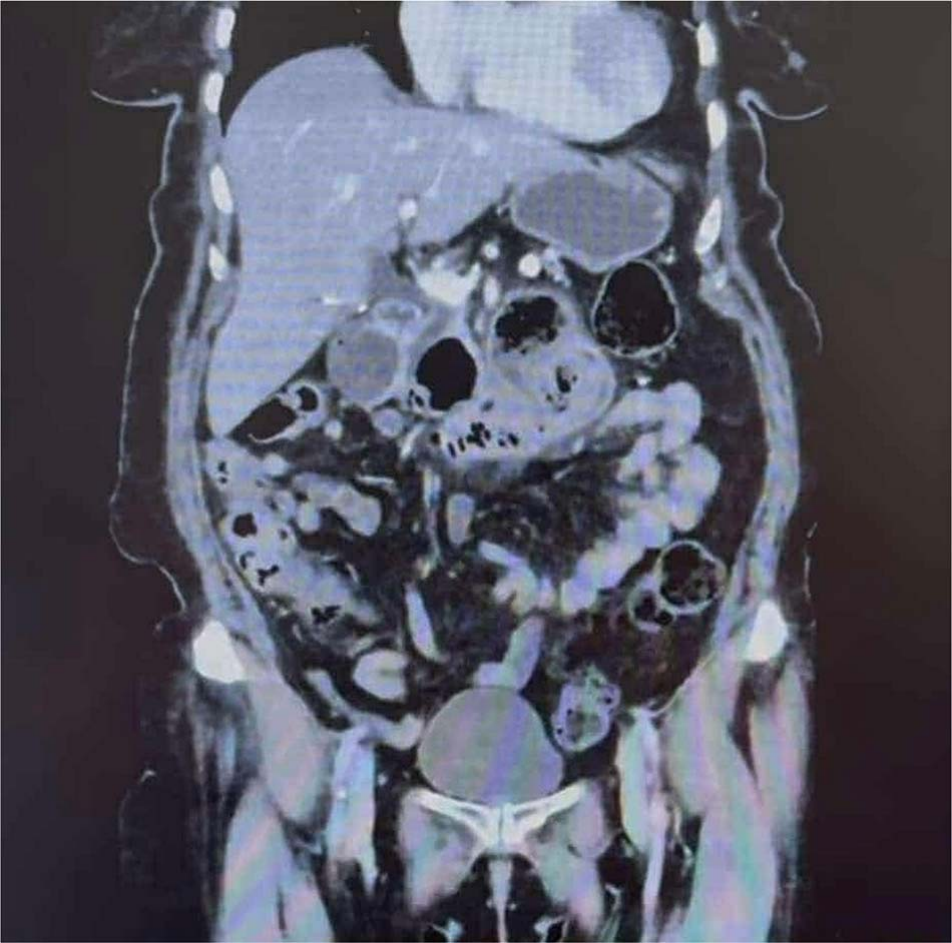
CT abdomen and pelvis demonstrating a partially obstructing intraluminal lesion at the duodeno-jejunal junction, adjacent to an inflamed duodenal diverticulum raising suspicion for an impacted food bolus, a mass lesion or intussusception. There was no evidence of perforation, free gas, or fluid.
